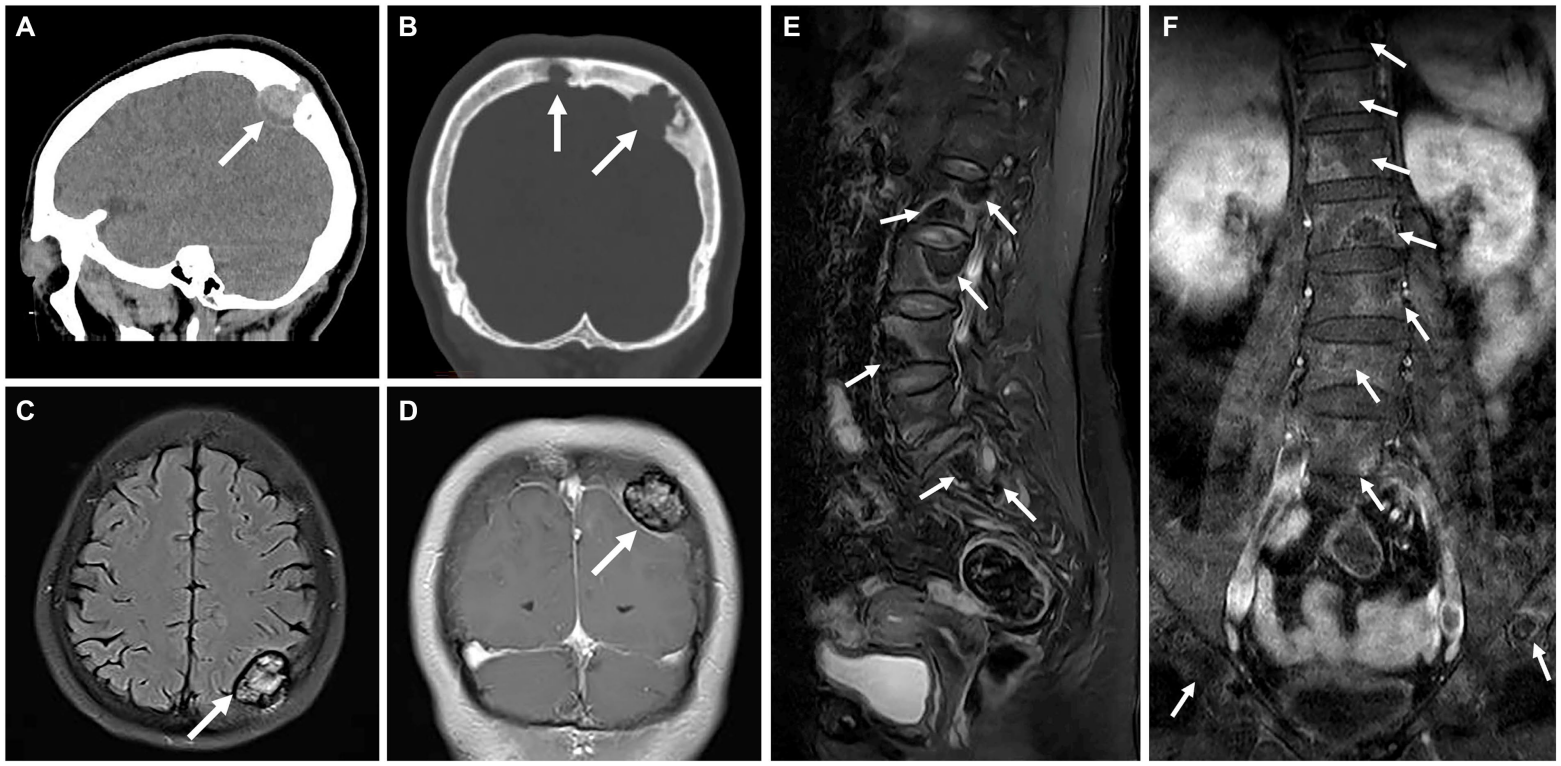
Computed tomography (CT) and magnetic resonance (MR) images of multicentric angiosarcomas of bone. Sagittal and coronal CT image revealed bone destruction of the parietal bone (A), accompanied by soft tissue lesions (long arrows), with the largest measuring approximately 3.6 × 2.7 × 2.0 cm, protruding into the epidural space (B). MRI examination demonstrated lesions with slightly elevated signal intensity on T2FLAIR (C, long arrows), showing moderate enhancement on Fat-sat Gd-T1WI (D). Multiple foci were observed within the T12, L1-5, and S1-2 vertebrae, as well as in the bilateral iliac bones. These exhibited irregular signal patterns on fat-suppressed T2WI (E, long arrows) and mild enhancement on Fat-sat Gd-T1WI (F).
